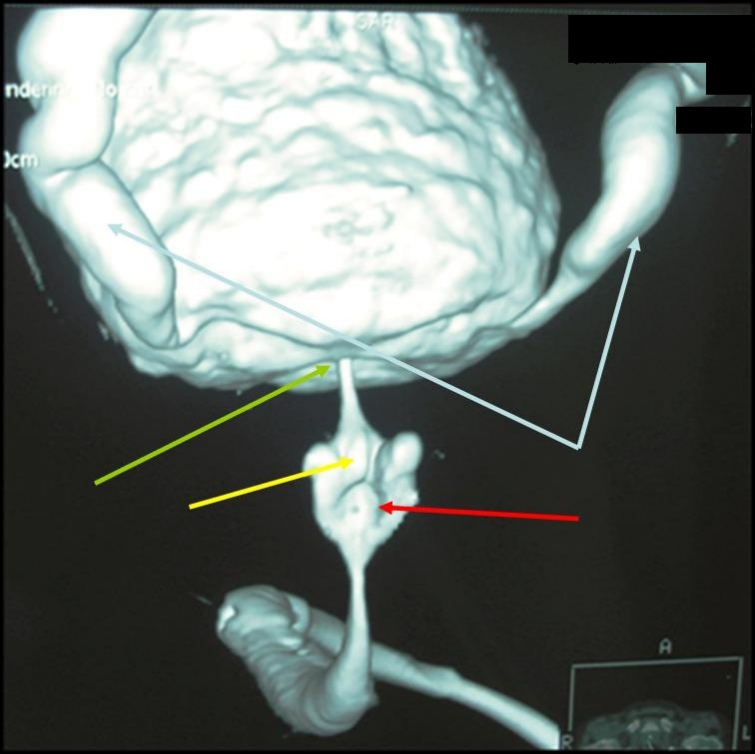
Three-dimensional computed tomography image. Three-dimensional computed tomography scan of a retrograde cystourethrogram reveals dilatation of the prostatic urethra (yellow arrow), a hypertrophic verumontanum (red arrow), bladder neck stenosis (green arrow), bladder distension with wall thickening, and vesicoureteral reflux with moderate ureter dilatation (blue arrows).
